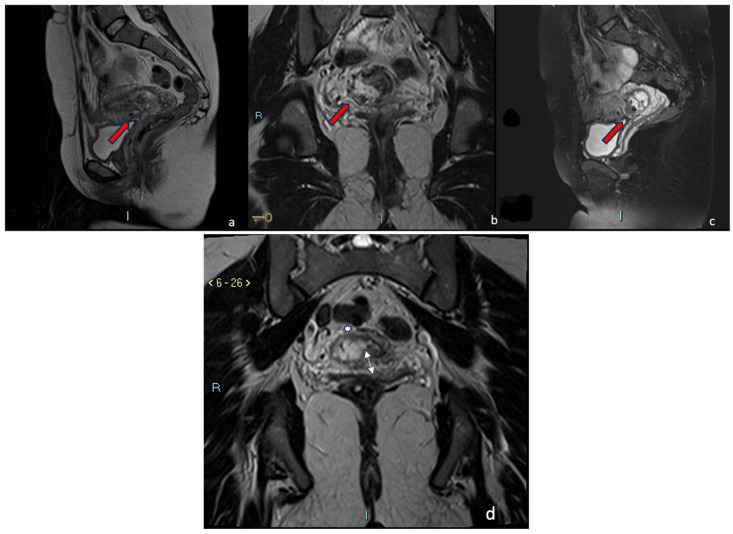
Low Implanted. Sagittal (a,c) T2-weighted and coronal T2-weighted fat-saturation (b) MRI sequences of the pelvis showed the gestational chamber implanted at the passage between the body and the neck of the uterus, near the previous cesarean section scar (red arrow). The serosa was focally interrupted throughout the myometrial wall, which was completely infiltrated. The posterior bladder wall did not show any evidence of invasion. (d) T2-weighted acquisition perpendicular to uterine cavity demonstrates implantation of the sac and internal uterine ostium with interruption of the rue internal uterine canal (white arrow) and the invasion of the myometrium (white star).
